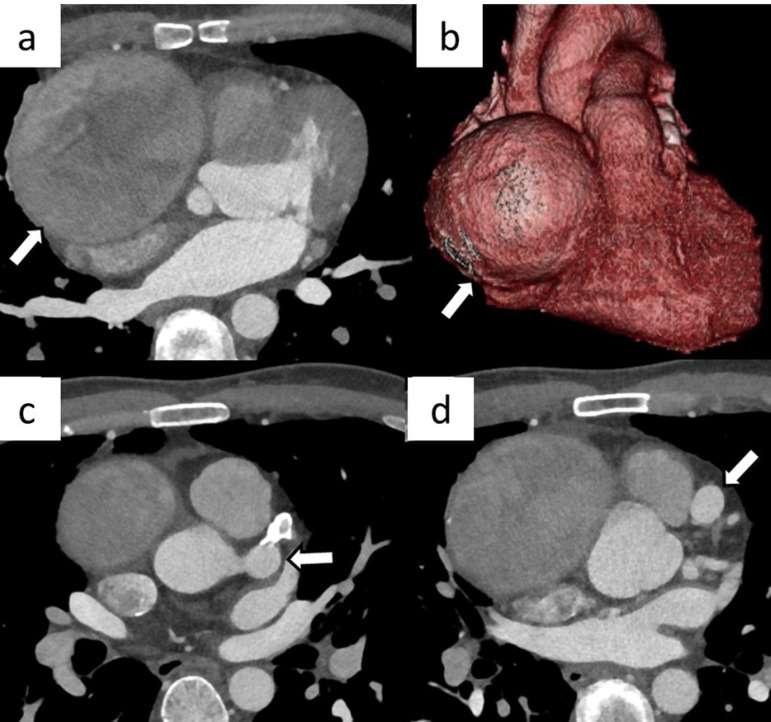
Preoperative cardiac computed tomography scan findings. a, b) Super-giant right coronary artery aneurysm (CAA) (88 mm) arising from the aortic root and displacing the right atrium (arrow). c) Giant CAA (14 mm) in the left main trunk (arrow). d) Giant CAA (12 mm) in the left anterior descending artery (arrow).
